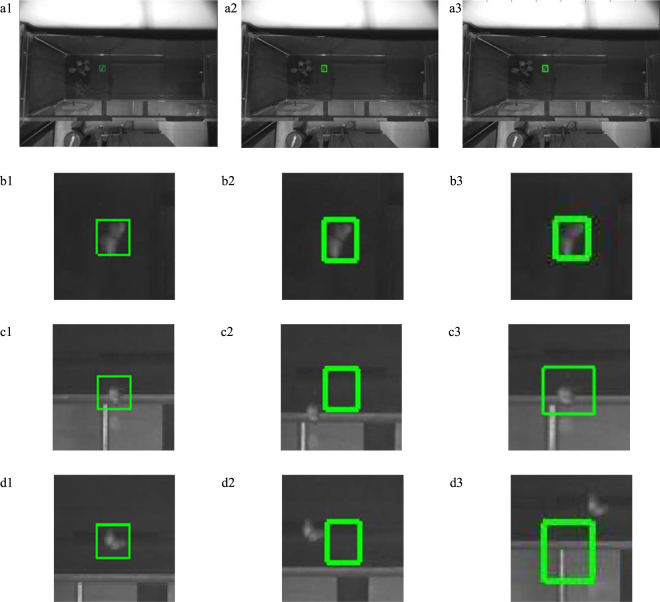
Comparison of objects using the KCF-BS algorithm (first column), CT algorithm (second column) and STC algorithm (third column). In all images, the rectangular box is the frame target tracking position. (a) First frame marking results. (b) Local map of the first frame. (c) Local map of 45th frame in the tracking process. (d) Local map of the 59th frame in the tracking process.
